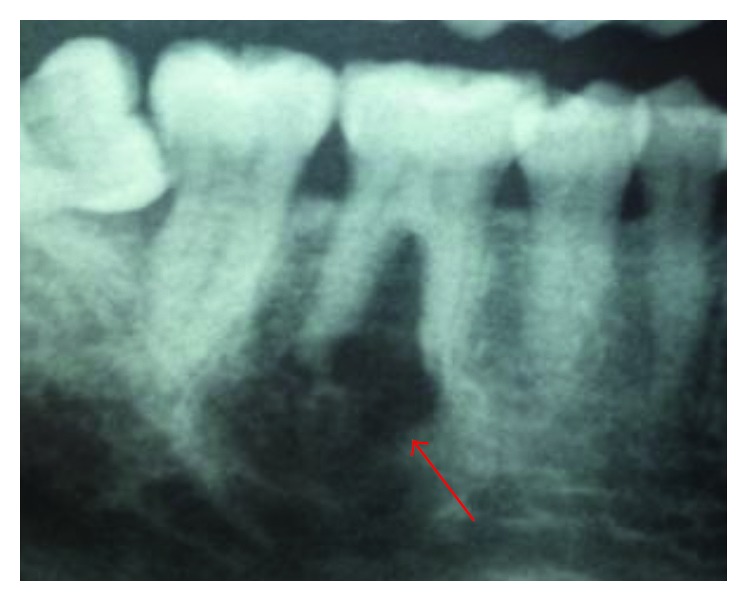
Panoramic radiography showing unilocular radiolucency close to the 46.
